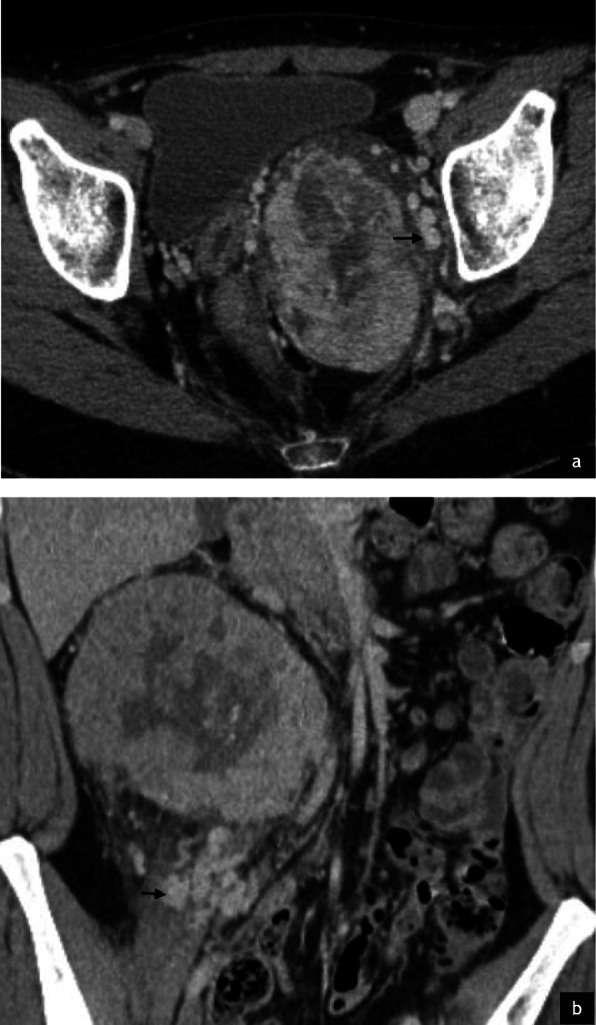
Primary ASPS within the pelvis in a pelvic 22 year old female (a) and intra-abdominal primary ASPS in a 24 year old male (b) patient. Post contrast axial (a) and coronal (b) CT demonstrates large tortuous feeding vessels at the periphery of both tumours (black arrows) with marked tumour hypervascularity. Although there is significant mass effect, both masses demonstrate well defined borders with no evidence of local invasion
